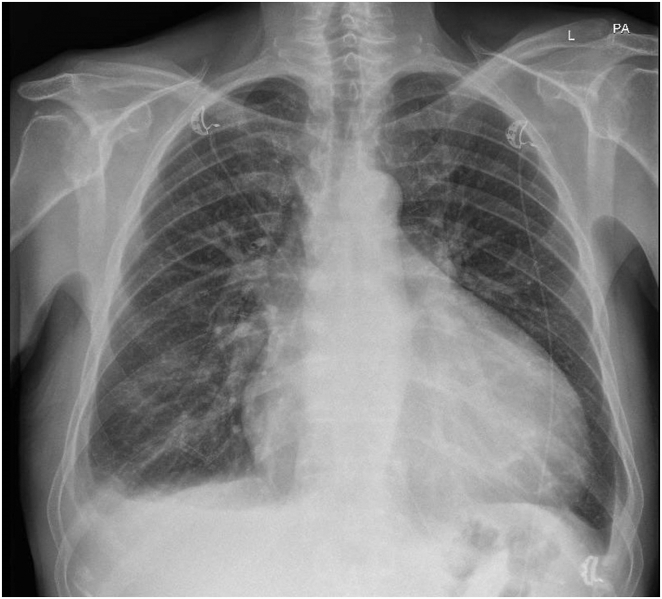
Chest radiograph showing cardiomegaly and right-sided pleural effusion.
